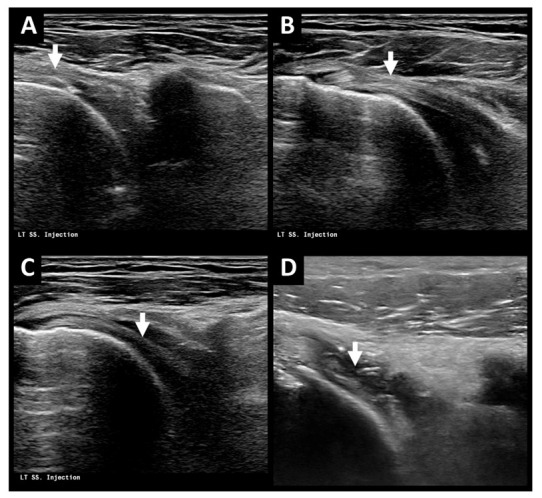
(A–C) Ultrasound images during ultrasound-guided hydrodilatation in a 69-year-old woman with AC of left shoulder via anterior rotator cuff interval approach with lateral in-plane view. Note visualization of the injection needle (white arrow, A), coracohumeral ligament (white arrow, B), and biceps tendon (white arrow, C) during needle placement. (D) Ultrasound image after ultrasound-guided hydrodilatation in a 54-year-old man with AC of left shoulder. Note the irregular and thickened glenohumeral joint capsule (white arrow) suggestive of capsulitis. AC = adhesive capsulitis.
